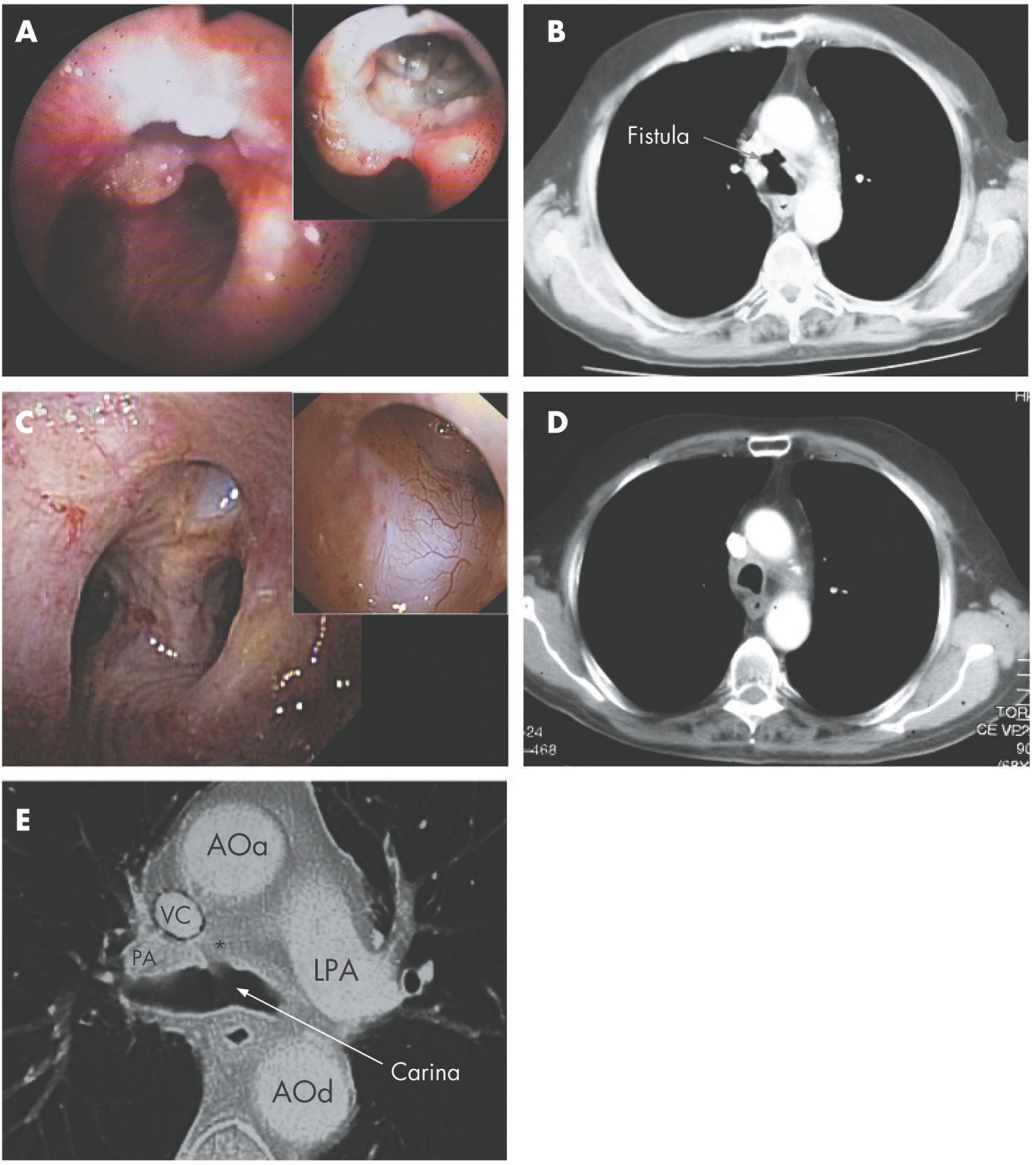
Bronchoscopic and CT images from the region of the fistula. a Bronchoscopic image recorded before cell therapy. The fistula can be seen on the anterior tracheal wall which had been totally destroyed after the laser treatment of the tumor. The entrance was about 10 mm in diameter and the bronchoscope could pass through it. Inset: Anthracotic mediastinal lymph nodes as seen through the wall of the fistula. b CT image recorded before cell therapy. The fistula was situated between the trachea and a pretracheal mediastinal cavity with an area of 2 cm^2^, next to the superior vena cava and pulmonary artery, near the ascending aorta. c Bronchoscopic image recorded 1 year after cell therapy. The entrance to the fistula was much smaller (diameter 3–4 mm). Inset: The walls of the fistula were covered with “new” epithelium and vessels as a result of neovascularisation and epithelialisation. d CT image from the same region of the fistula 1 year after cell therapy. One year after treatment the cavity had disappeared. e CT image from the region of the fistula recorded 1 year after cell therapy. This image is the only one to show remnants of the previous fistulous tract. It is clear that the fistula had closed. *, small depression; VC, superior vena cava; AOa, ascending aorta; AOd, descending aorta; PA, right pulmonary artery; LPA, left pulmonary artery. Reproduced with permission from Díaz-Agero Álvarez et al. [38]
